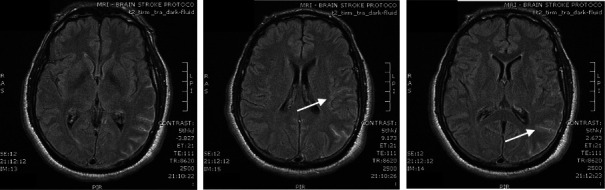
Brain magnetic resonance images show abnormal hyperintensity at the sulci in the left temporoparietal region and at the left side of the ambient cistern (arrows), raising the suspicion of subarachnoid hemorrhage.
